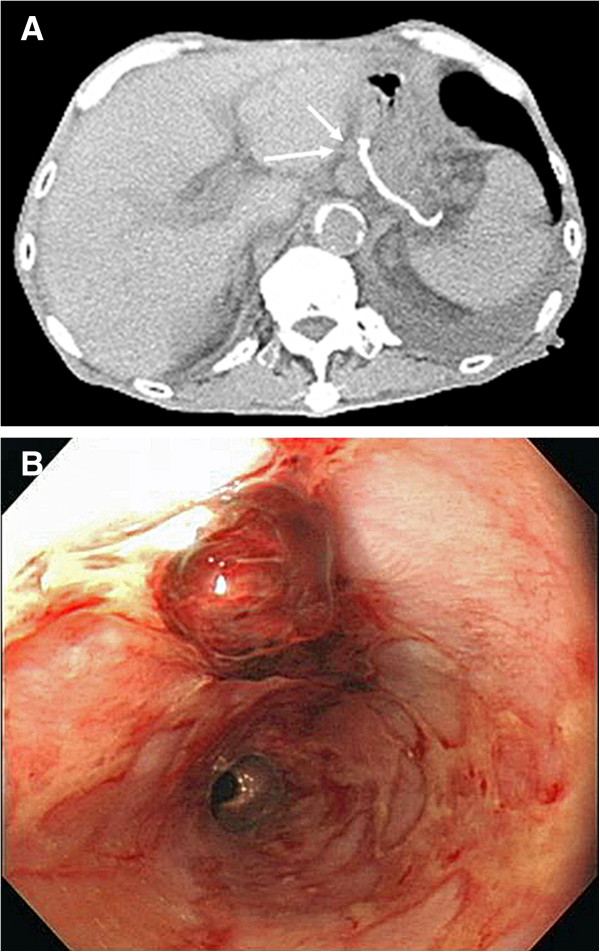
Postoperative course Postoperative course. Five months after the last course of chemotherapy, computed tomography (CT) scans revealed a recurrent regional lymph node (arrow) but no recurrence in the liver (A). A contemporary gastrointestinal endoscopy revealed hemorrhage from an esophagus longitudinal ulcer, but no carcinoma recurrence was apparent in the esophagus (B).
